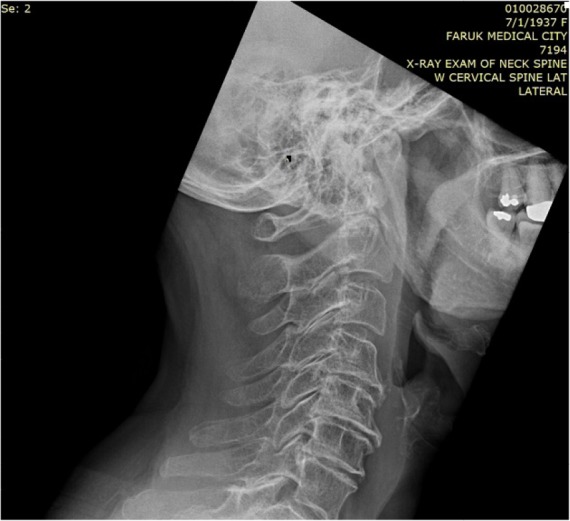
Cervical radiograph: spondylosis.
